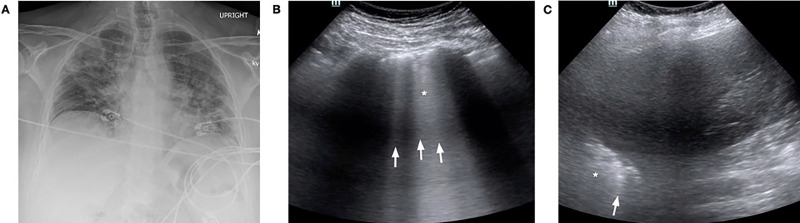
Case 3 imaging findings: (A) Anterior-posterior chest radiograph at the time of presentation to the emergency department demonstrating diffuse peripherally-based bilateral patchy opacities. Lung point-of-care ultrasound in the emergency department was remarkable for confluent B-lines posterior (B, arrows), as well as an irregular pleural line with subpleural consolidations (asterisk). A lateral view (C) in the mid-anterior axillary line showed similar B-lines (arrow) and an irregular pleural line (asterisk).
